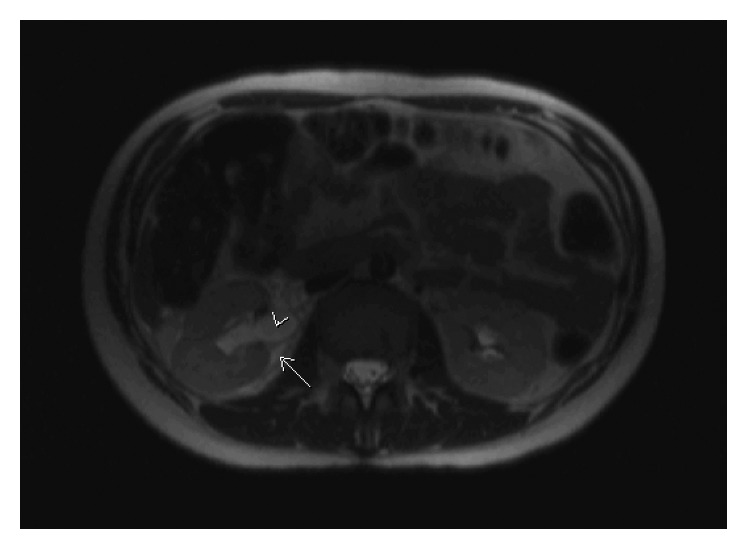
Coronal slice of MRI obtained on admission showing right hydroureter (arrowhead) and fluid extravasation surrounding the right kidney (arrow).
